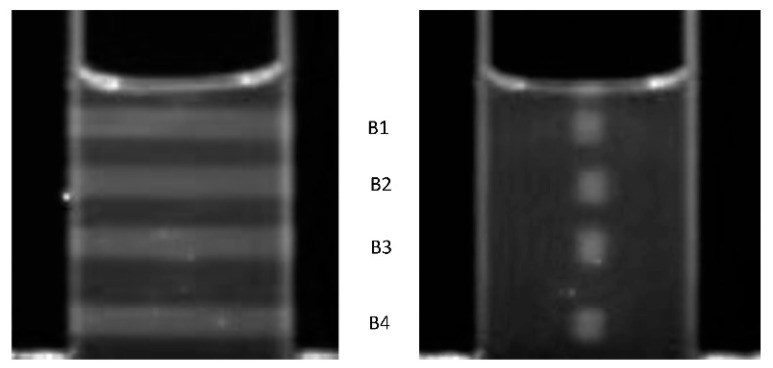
Images taken with Fluorotome 1 of a 20 × 20 mm square, ±30 mm long RFG gel that has been irradiated with four 3 mm square 300 kVp x-ray beams, denoted B1-B4 in the figure. All have total entrance doses of 15.9 ± 0.3 Gy with dose rates from 3.9 to 1.3 Gy/min (top to bottom). The plane of the UV-sheet was at the center of the cell (depth 10 mm) and parallel (left) or orthogonal (right) to the x-ray penetration direction.
